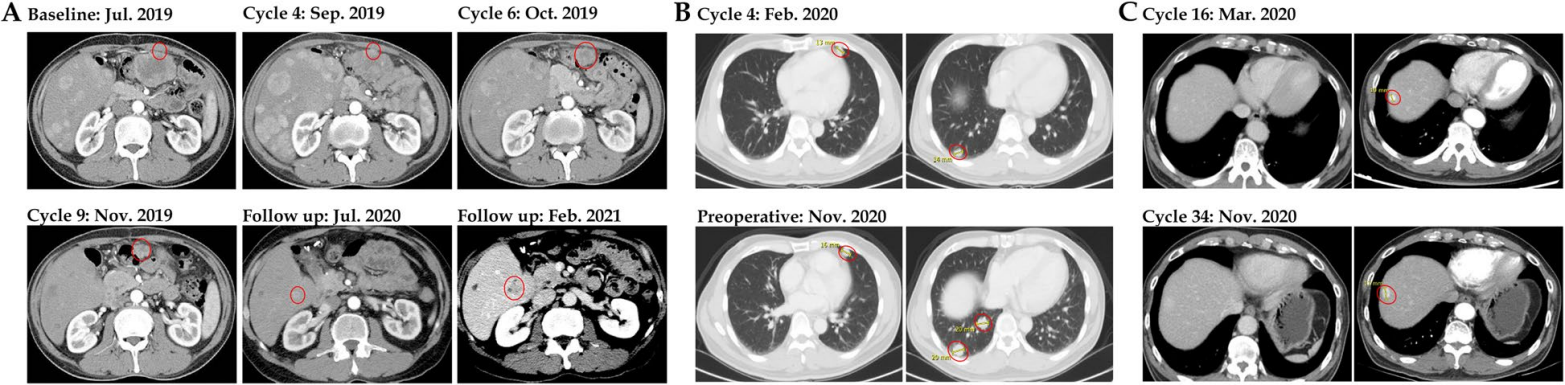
CT images of representative cases. A Patient 2 experienced pseudo-progression after 4 cycles of GT90001 plus nivolumab treatment and achieved partial response at cycle 6. B Patient 6 with lung metastases achieved stable disease under treatment while also experiencing progressive enlargement of lung lesions in February 2020. After surgical resection for lung lesions in November 2020, the patient remained recurrence-free for more than 6 months. C Patient 1 with lung metastases achieved PR under the treatment but experienced HCC recurrence
